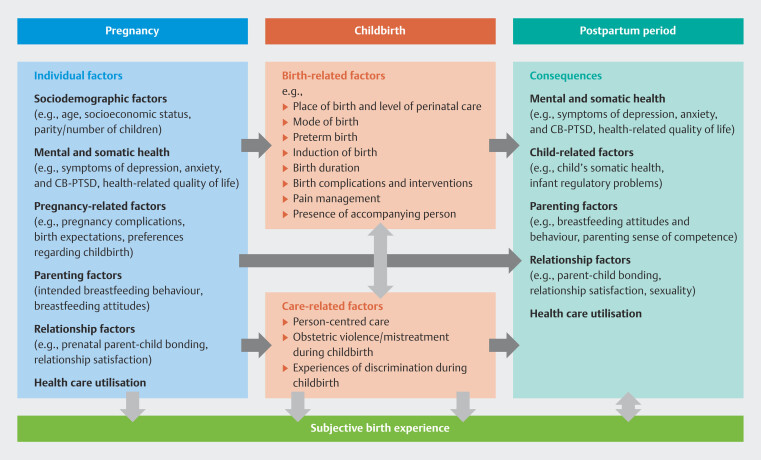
Simplified theoretical framework upon which the main study RESPECT
